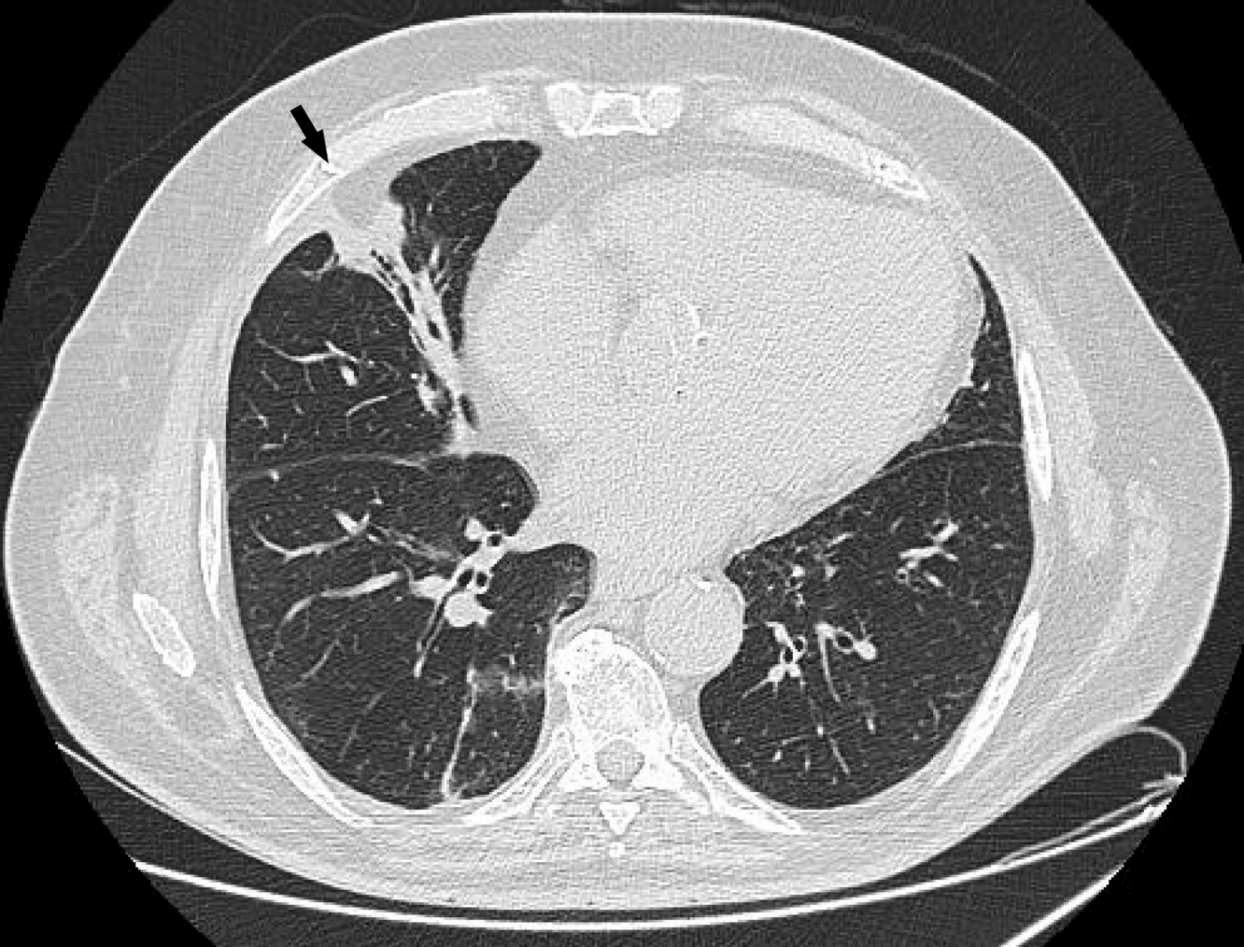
CT scan showing complete resolution of the necrotizing pneumonia, with only residual scarring remaining (indicated by the arrow).
